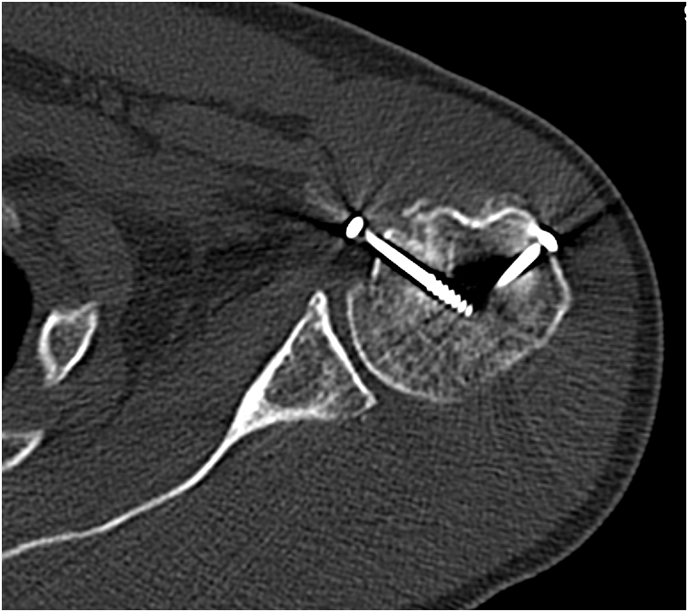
Transverse CT showing screw migration 2 months after the surgery in a 46-year-old man, with the need for surgical removal.
